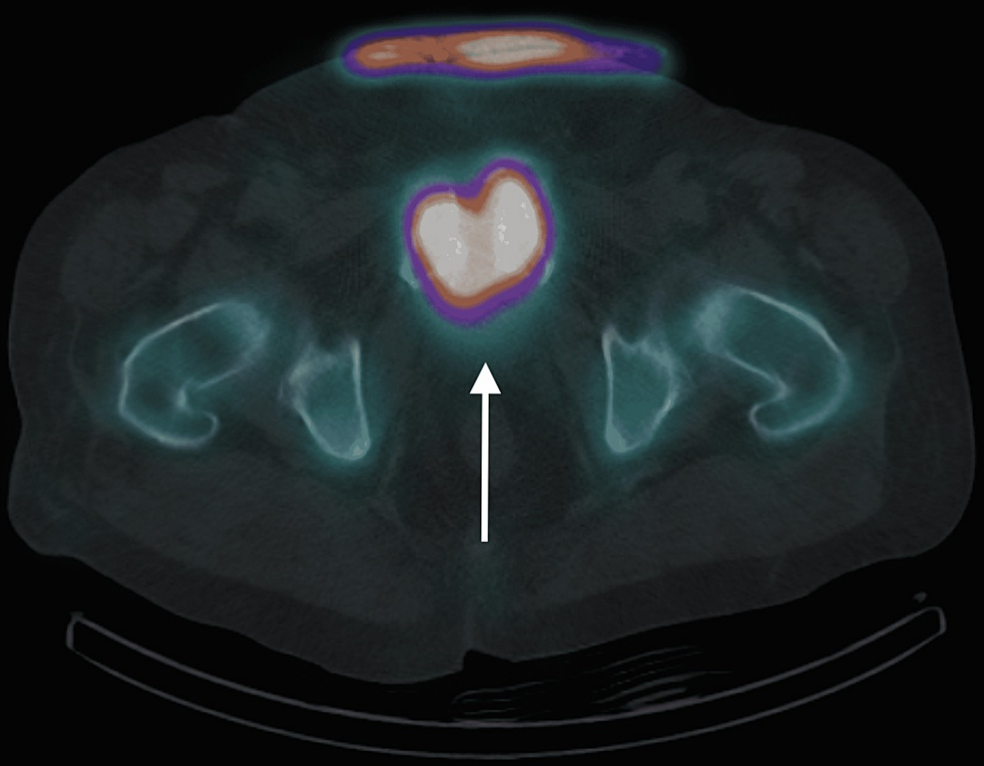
Bone scan SPECT/CT. Bone scan SPECT/CT axial image shows intense uptake in the anterior pubic bones. SPECT/CT: single-photon emission computed tomography/computed tomography
